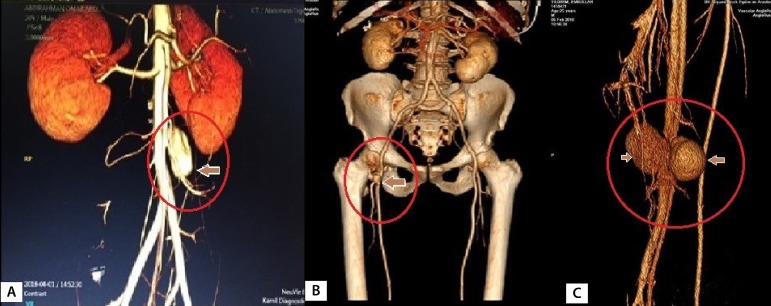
A) Fistulization of supra-popliteal arterial pseudoaneurysm to the popliteal vein in a patient with BD and history of coronary artery bypass (35-year-old female patient with no history of trauma); B) CT angiographic appearance of pseudoaneurysm formation at the right common femoral artery access site (39-year-old male patient); C) In a case with BD, the infrarenal saccular aneurysm in the abdominal aorta (55-year-old male patient; the lesion was stented before CABG).
